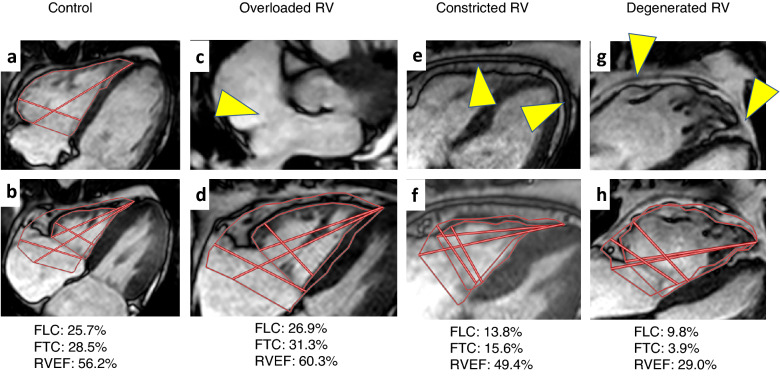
Representative cases. The straight red lines indicate the measured locations for RVLd, RVLs, RVDd, and RVDs. The polygonal lines indicate the endocardium of the RV at end-diastole and end-systole. (a, b) Four-chamber cine CMR of a woman in her 40 s; (a) end-diastole; (b) end-systole. CMR revealed no morphological abnormalities in the RV. (c, d) A woman in her 40 s with a history of ventricular tachycardia. CMR revealed an atrial septal defect and a left-to-right shunt (c, arrow). The Qp/Qs ratio was estimated at 2.2 by the phase-contrast method. (e, f) A man in his 70 s with right heart failure. Thickened pericardium is visible (e, arrow). (g, h) A man in his 40 s with a history of ventricular tachycardia. Remarkable impairment of RV contraction, and bulging and fat infiltration of the RV (g, arrow) are visible. *RVLd* Right ventricular length at end-diastole, *RVLs* Right ventricular length at end-systole, *RVDd* Right ventricular diameter at end-diastole, *RVDs* Right ventricular diameter at end-systole, *CMR* Cardiac magnetic resonance imaging.
